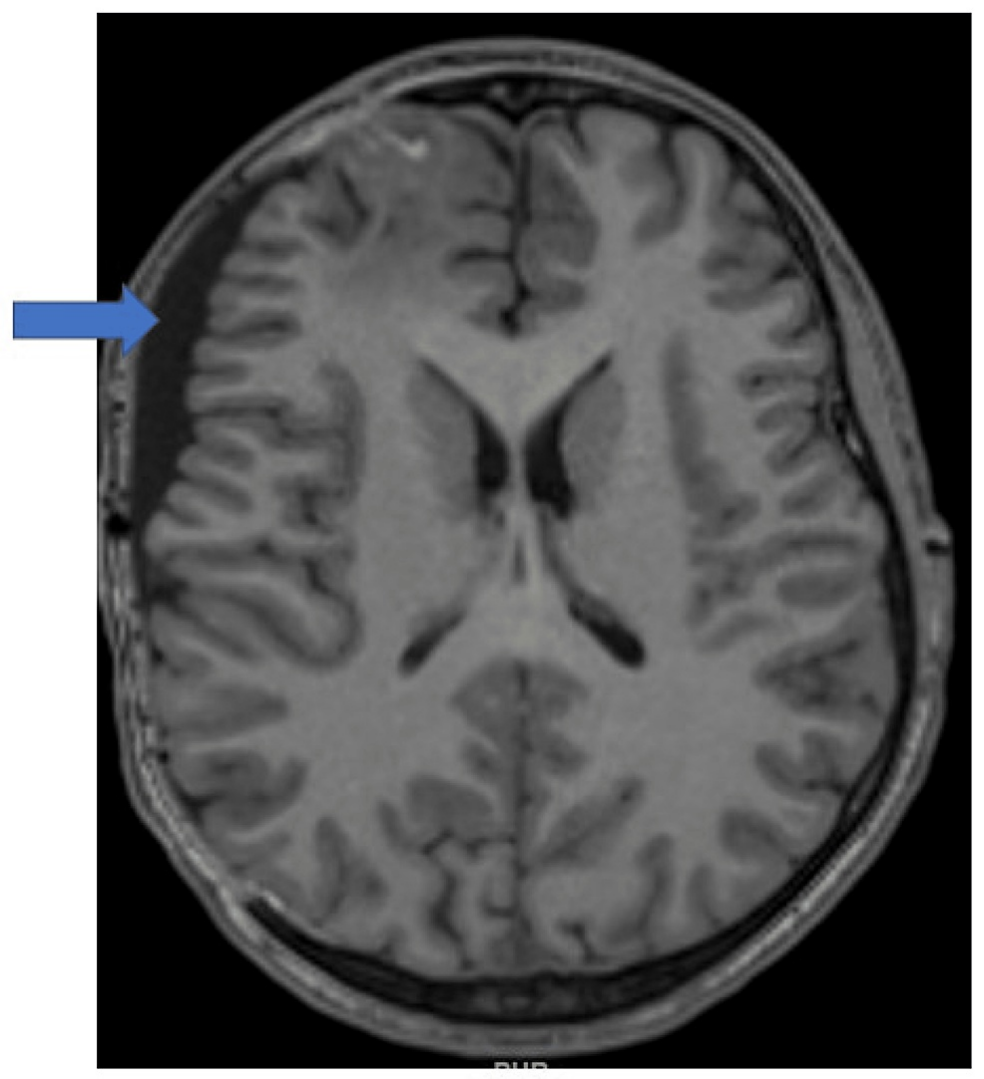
Repeat MRI of the brain at five weeks post-discharge with the craniectomy flap (arrow). MRI: magnetic resonance imaging.
